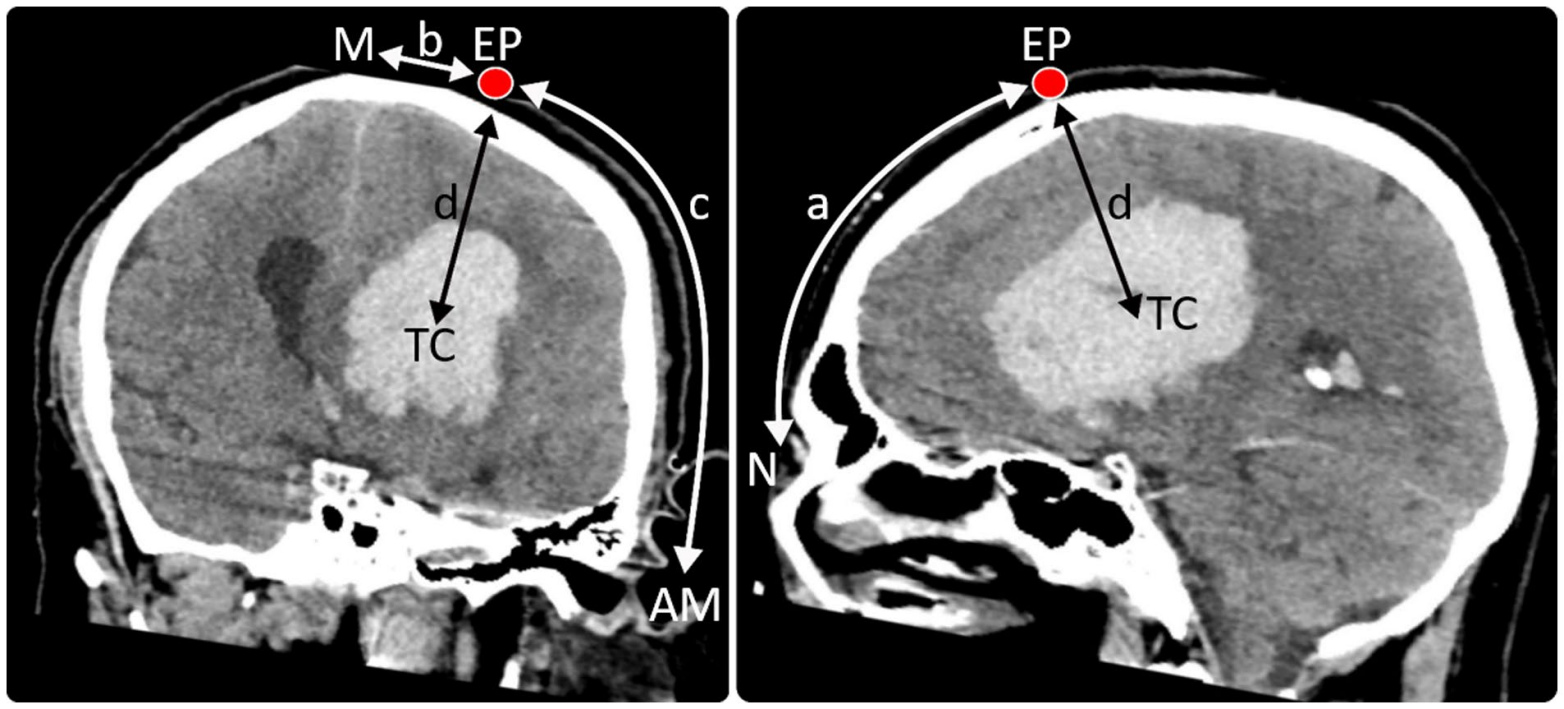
Entry point (EP) and trajectory of the catheter were planned using a 3D reconstructed CT scan. The modified technique described by Seeger, 1980 (*) was used to determine the distances of the entry point (EP) from the nasion (N) (distance a), midline (M) (distance b), and acoustic meatus (AM) (distance c) as well as the distance between the skin level to the target position of the tip of the catheter (TC) within the haematoma (distance d) in the 3D image. These coordinates were then transferred to the patient’s head. (*) Microsurgery of the Brain: Anatomical and Technical Principles. Author: Prof. Dr. med. Wolfgang Seeger (auth.); Publisher: Springer Vienna, Year: 1980; pp. 46–53.
